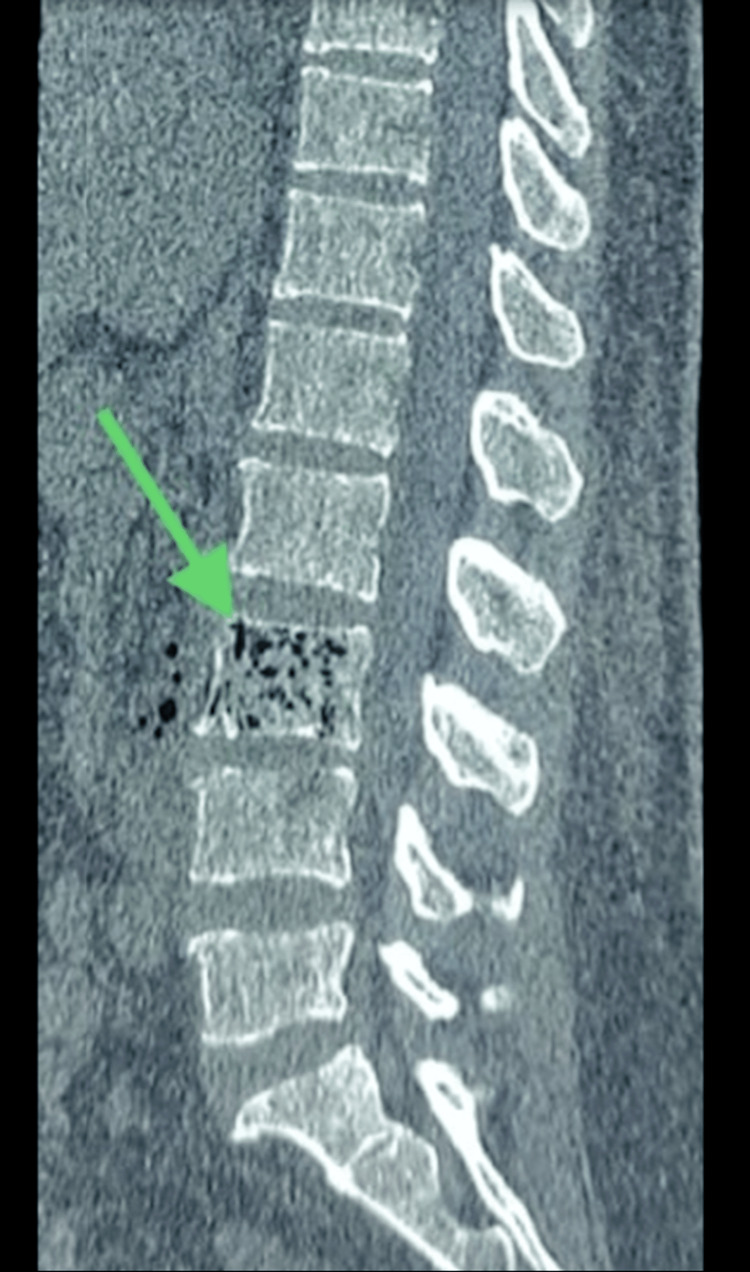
CT scan of the spine in the sagittal plane view shows intraosseous air foci at the L3, indicated by the arrow. CT: computed tomography, L3: third lumbar spine vertebra
